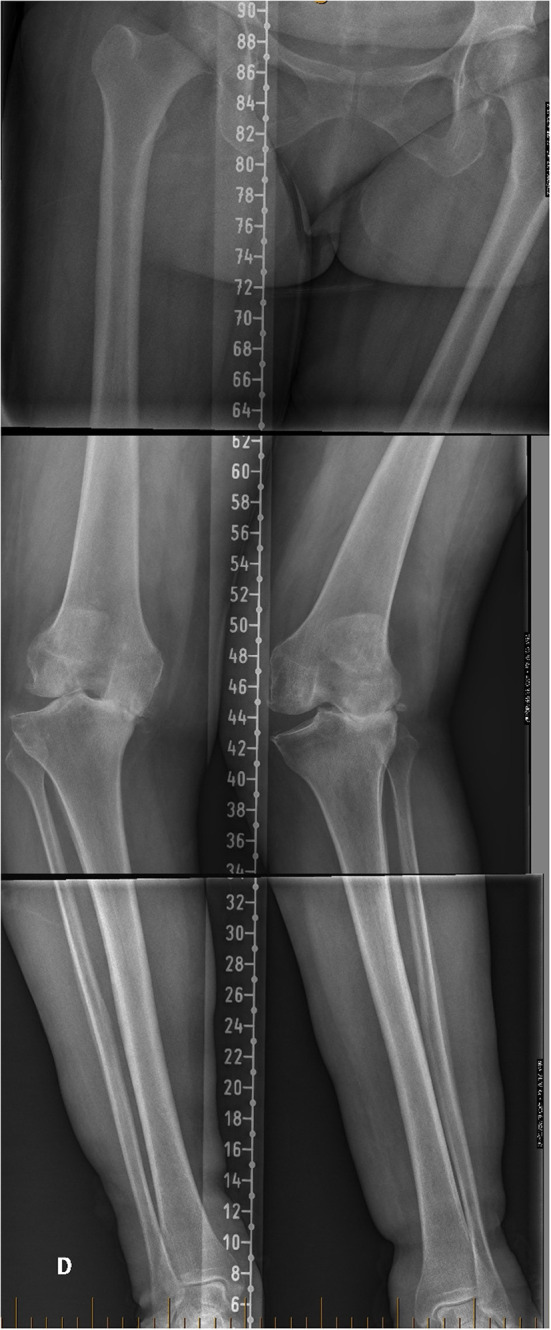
Lower limb full-length radiographs showing lower limbs with windswept deformity. Her mechanical distal femoral angle (mDFA) is 10° valgus on the right femur vs. 1° on the left side. Regarding tibial anatomy, her right mechanical proximal tibial angle (mPTA) is at 0° vs. 5° varus on the left. Because of her important lower limb asymmetry, we consider her lower limb anatomy to be pathologic. Applying unrestricted KA technique would reproduce her lower limbs malalignment.
